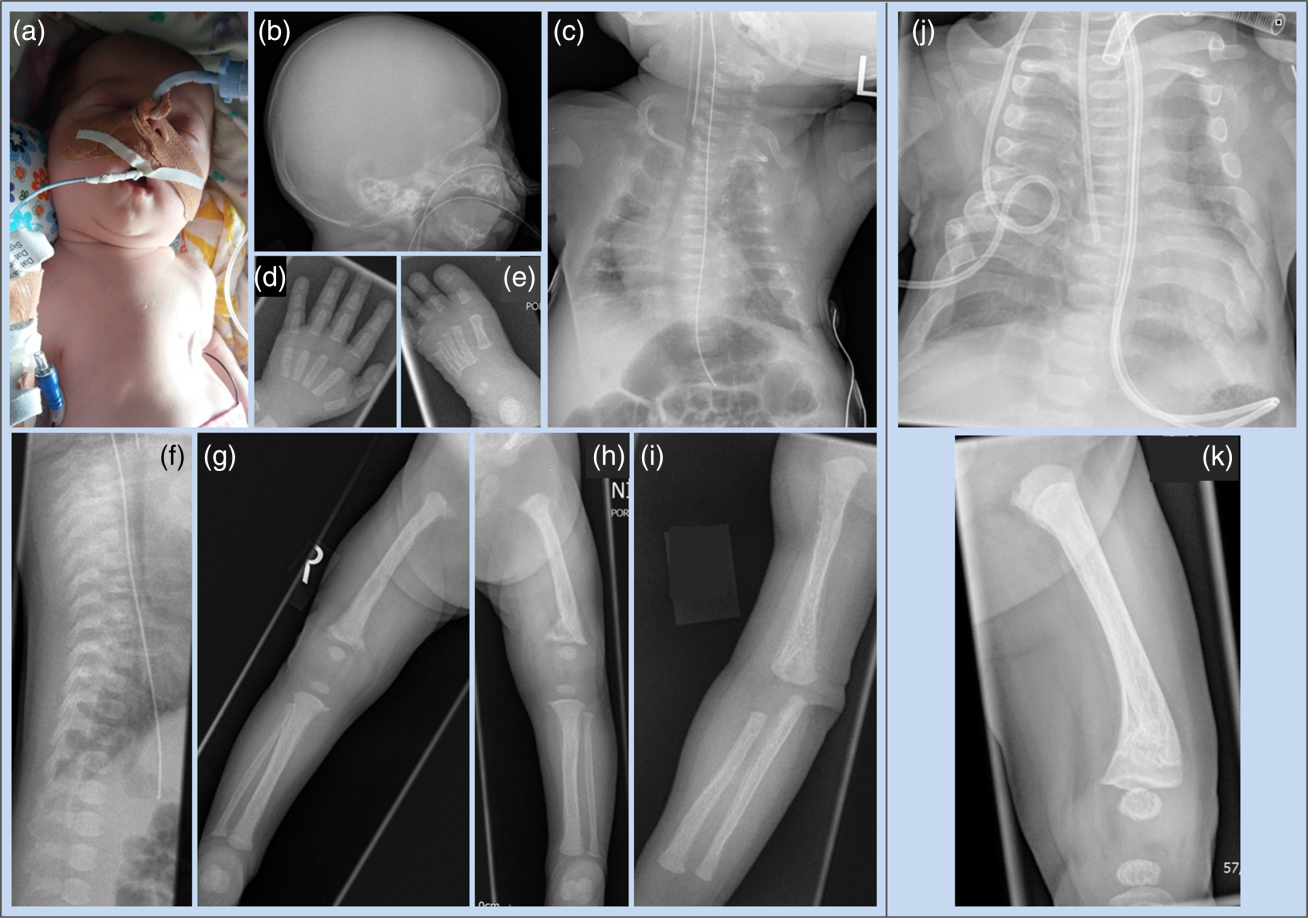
Clinical and radiological findings. (a) The bell‐shaped chest was associated with respiratory distress. (b–i) The skeletal survey at the age of 2 weeks showed generalized undermineralization, short, thin, and fractured ribs, absence of Wormian bones and normal vertebrae. The long bones showed a similar pattern of metaphyseal irregularities with corner fractures and periosteal reaction most obvious along the diaphyses of femora, tibiae and humeri. (j, k) Radiographs of chest and femur at the age of 10 weeks, showed broader, longer ribs, partial resolution of the metaphyseal lesions, and improved bone mineralization [Color figure can be viewed at wileyonlinelibrary.com]
